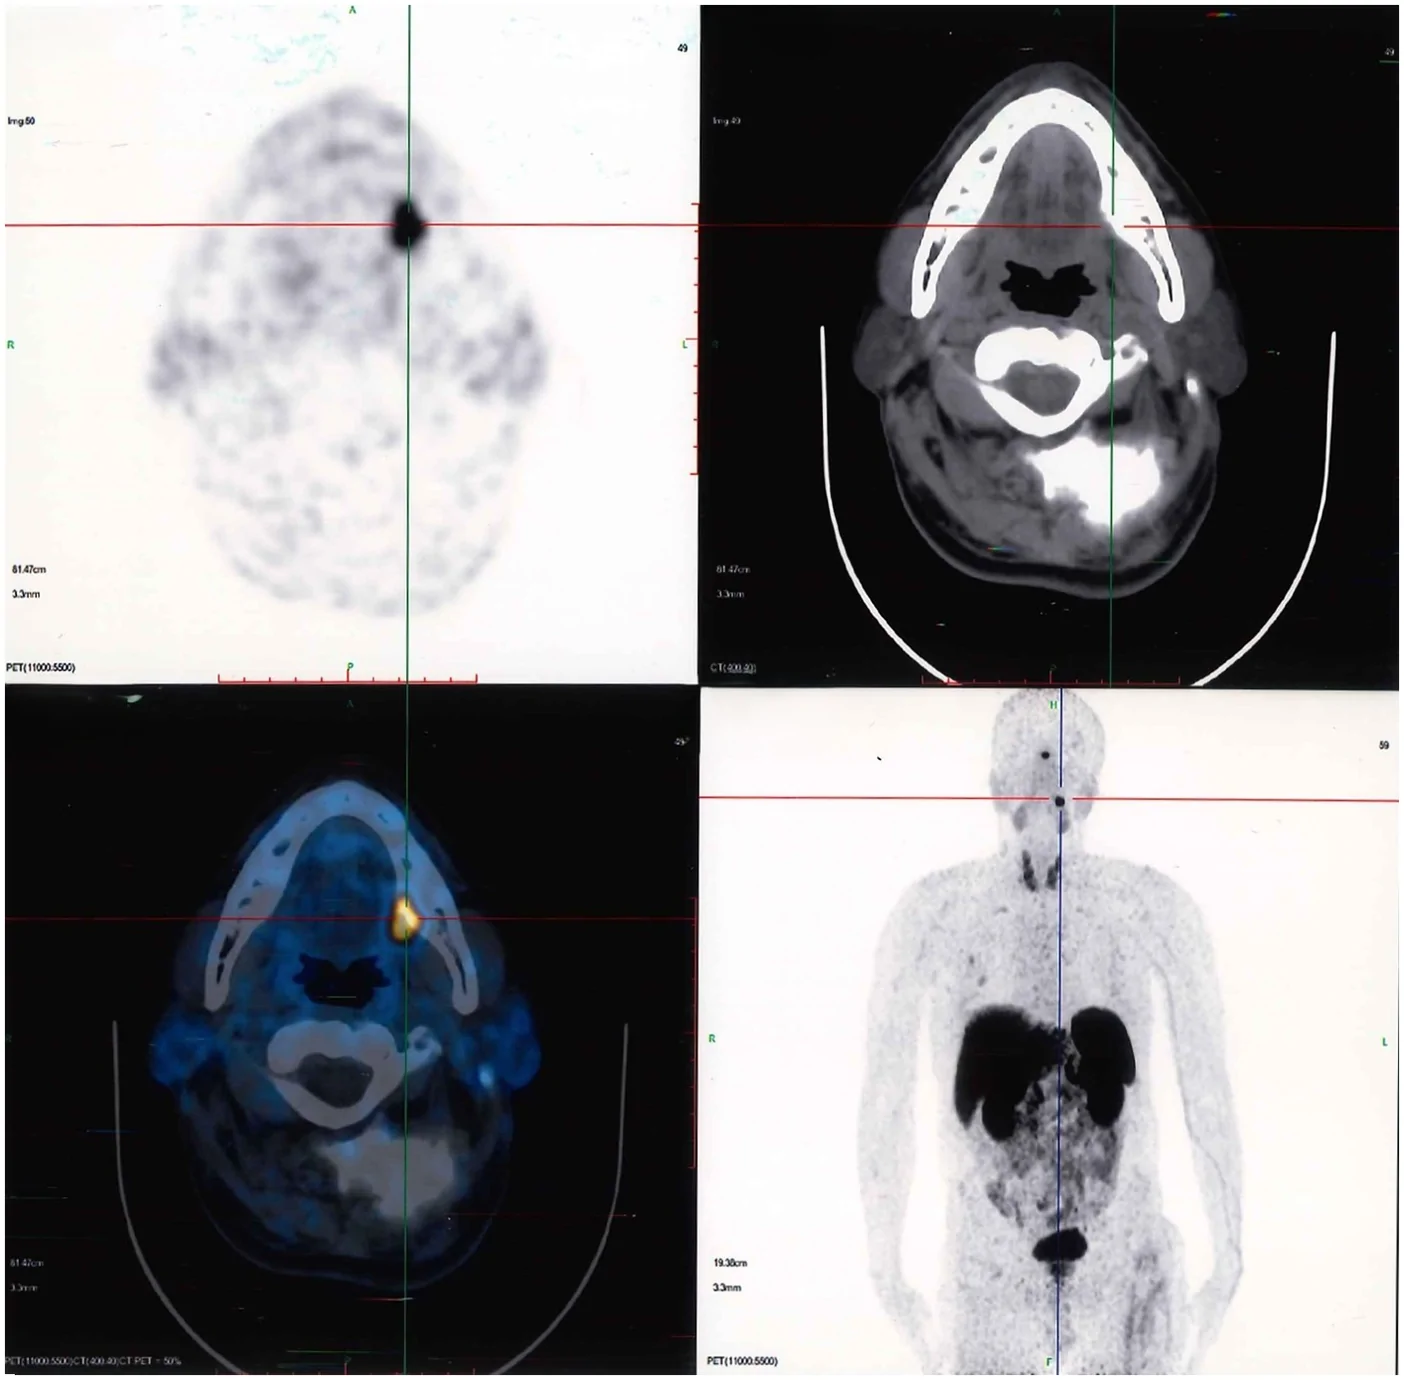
^68^Ga-DOTATATE PET/CT showed focal radiotracer uptake in the left mandibular lesion (lesion SUVmax = 10.3), accompanied by a low-density nodular lesion in the bone tissue and localized enlargement in the medial region of the left molar bone.
